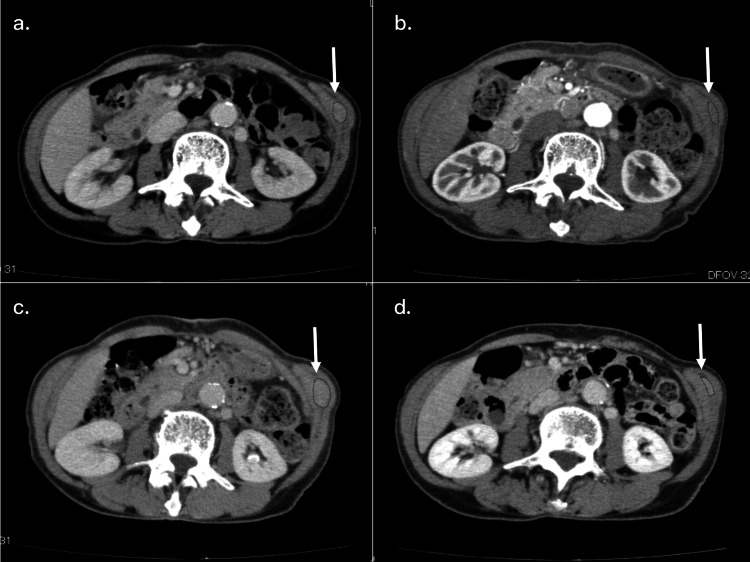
Sequential CT findings of the recurrent abdominal wall tumor (arrows and dotted areas) (a) The tumor in the left lateral abdominal wall was initially maintained as SD for 12 months after starting first-line chemotherapy but later became PD. The tumor was maintained as SD for 11 months after the introduction of second-line chemotherapy (b) but became PD thereafter (c). (d) Subsequently, third-line chemotherapy using nal-IRI + FF was introduced, and the tumor was maintained as SD for 12 months with an excellent physical condition. CT: Computed tomography; SD: stable disease; PD: progressive disease; nal-IRI + FF: nanoliposomal irinotecan plus fluorouracil and folinic acid.
